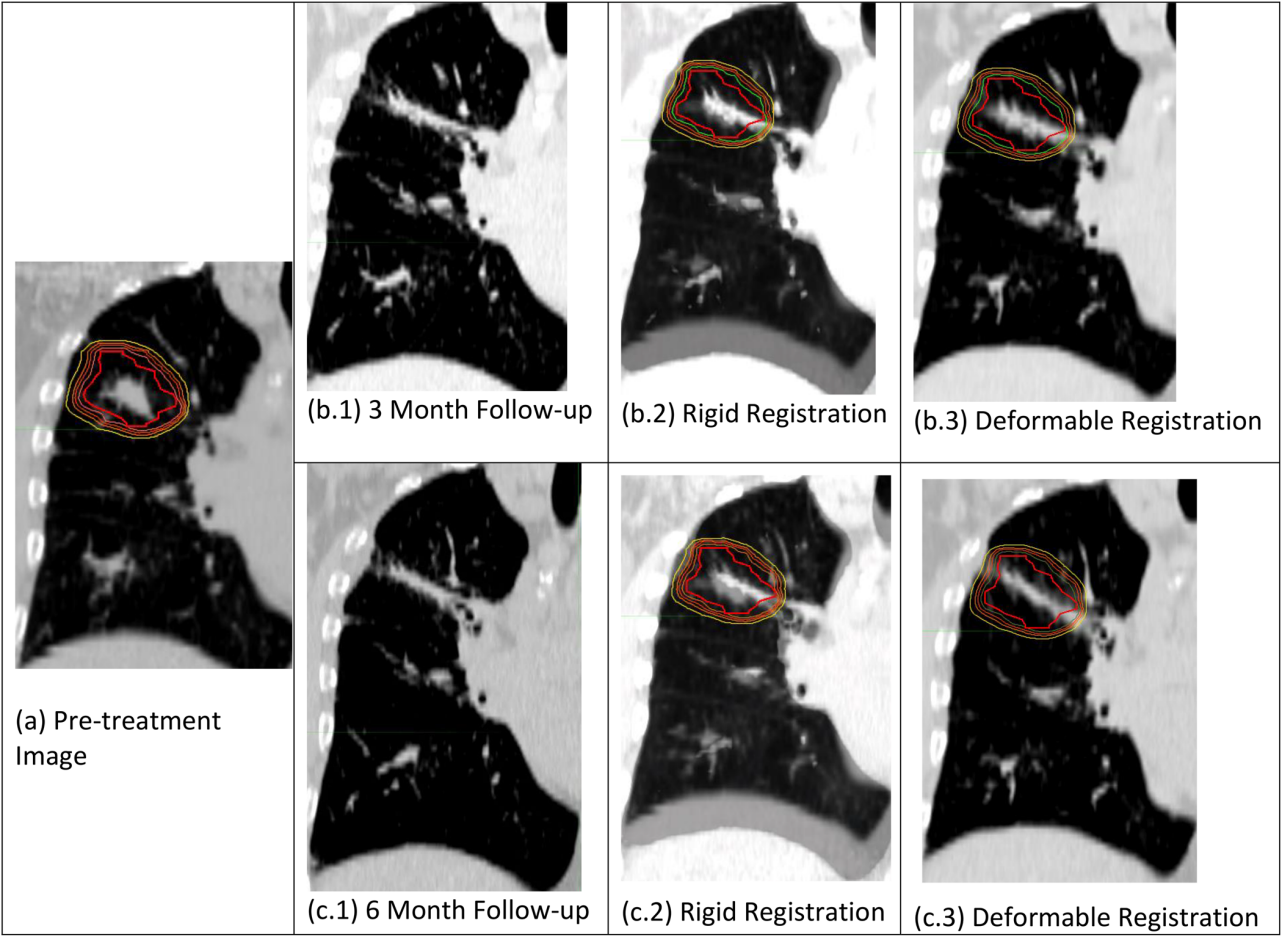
Example of image registration. (a) Baseline pre‐treatment (used for treatment planning) CT scan (coronal section) with the PTV (red) and isodose lines. (b.1) 3‐month follow‐up CT scan. (b.2) Rigid registration with pre‐treatment CT scan and 3 month follow‐CT scan. (b.3) Deformable registration with pre‐treatment CT scan and 3‐month follow‐up CT scan with PTV volume and isodose curves. (c.1) 6‐month follow‐up CT scan. (c.2) Rigid registration with pre‐treatment CT scan and 6 month follow‐CT scan. (c.3) Deformable registration with pre‐treatment CT scan and 6‐month follow‐up CT scan with PTV volume and isodose curves
